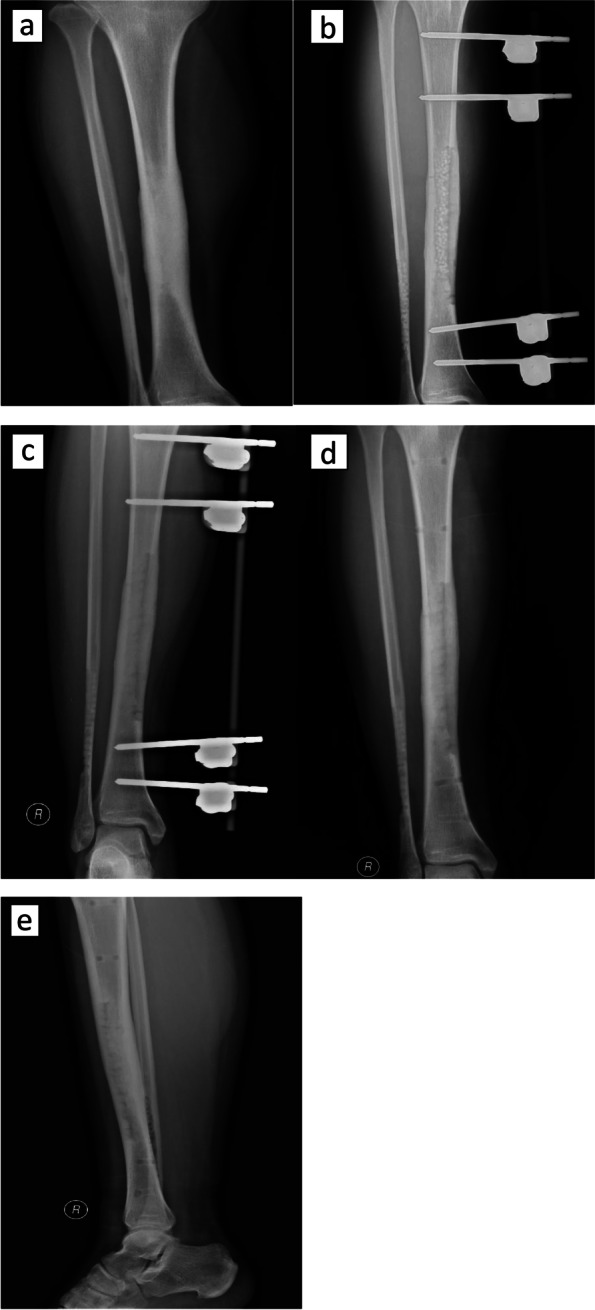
Typical case. A 31-year-old male patient presented with right leg pain that worsened at night. a Preoperative X-rays showed thickening and hardening of the middle and lower tibia, and narrowing of the medullary cavity. b Twenty days after the operation, the density of the medullary cavity of the middle and lower tibia and the lower fibula was reduced, and there was a granular high-density shadow filling. c Two months after the operation. d Five months after the operation, the external fixator was removed. e Seven months after the operation.
